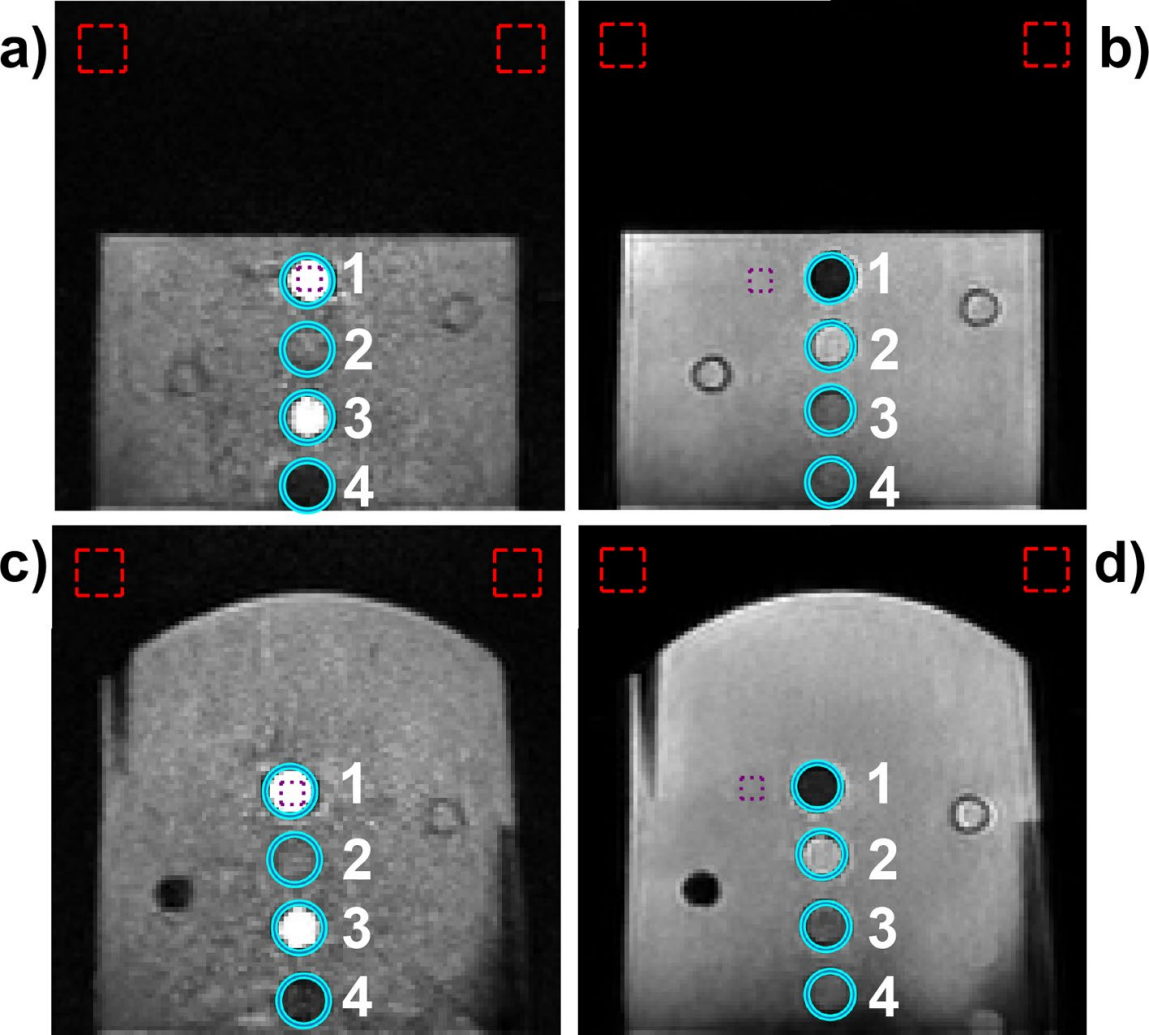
64 mT T1-weighted (a, c) and T2-weighted (b, d) images for flat top (a, b) and domed top (c, d) coronal plate acquisitions. Geometric distortion was calculated for each image using the distance between the center of consecutive ROIs (cyan circles, numbers indicate consecutive ROIs). Rectangles mark two noise regions (red) and one signal region (purple) used for SNR and CNR calculations
